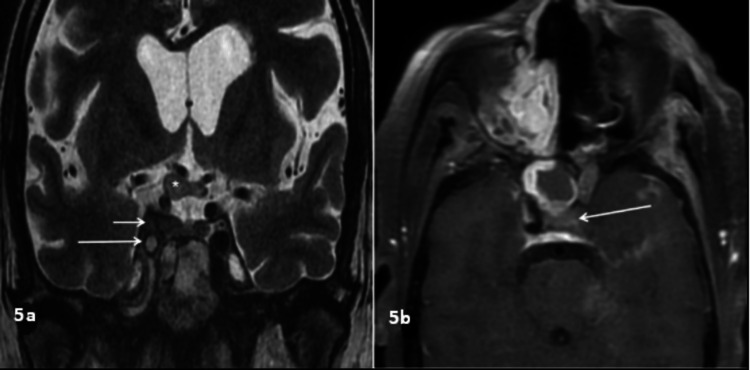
Cavernous sinus involvement 5a: Coronal T2WI of a 42-year-old patient who had recent COVID-19 and systemic steroid use who presented with headache and right eye vision loss showing loss of flow of void in the right ICA (large arrow) with abnormal signal intensity in the right cavernous sinus surrounding the right ICA (small arrow) and thickening of the right optic nerve and optic chiasma (asterisk). 5b: Axial post-contrast FS T1WI of a 50-year-old patient who had recent COVID-19 and systemic steroid use who presented with headache and left eye vision loss showing loss of flow void in the left ICA (arrow). T2WI: T2-weighted image, COVID-19: coronavirus disease 2019, ICA: internal carotid artery, FS T1WI: fat-suppressed T1-weighted image
